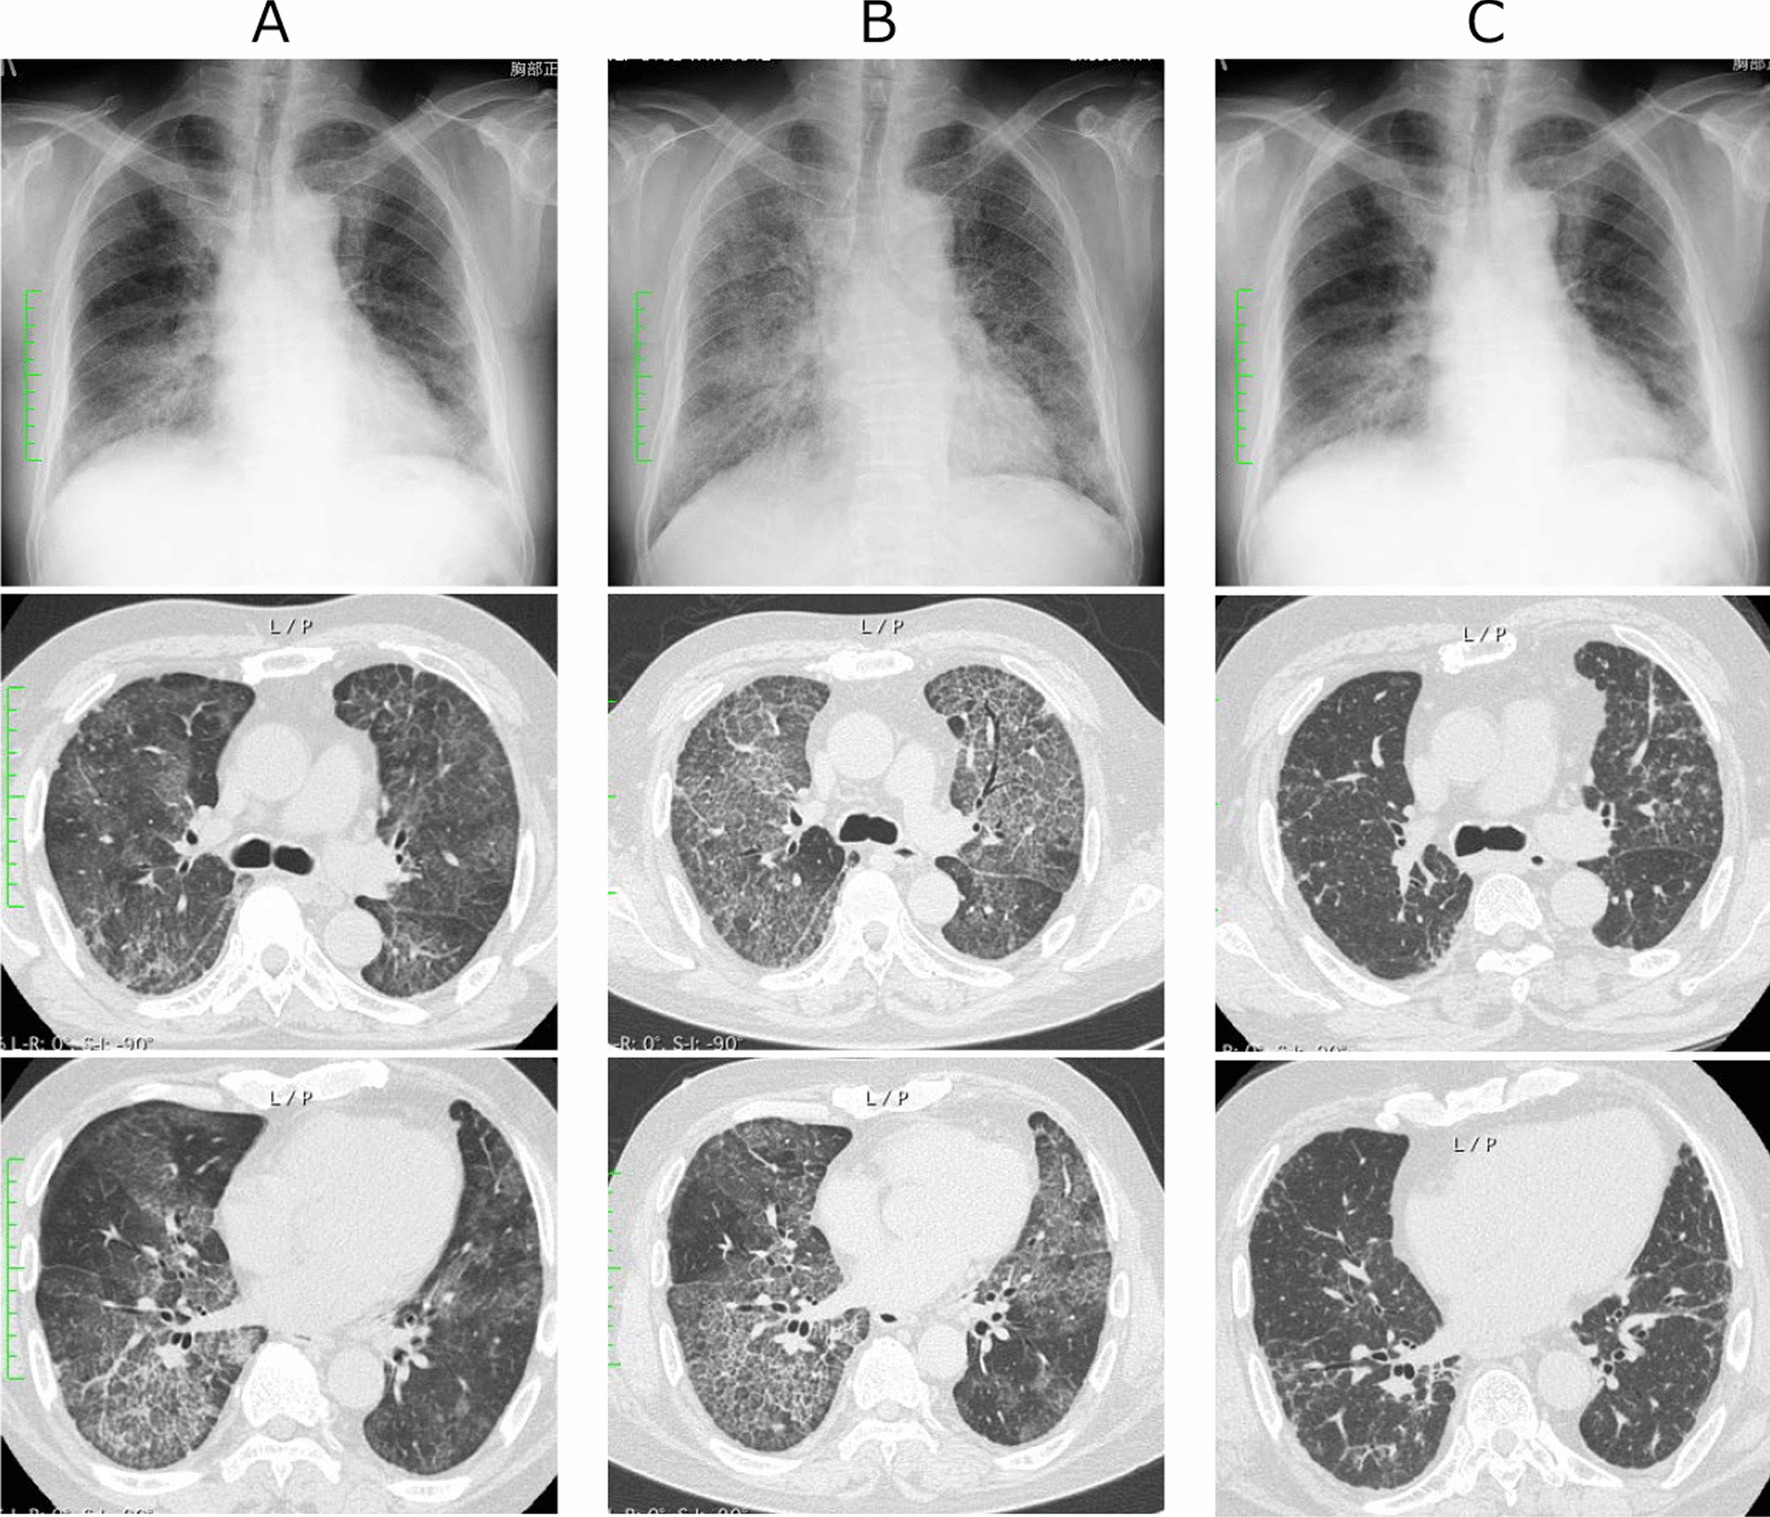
Chest radiography and computed tomography findings. First visit to the previous hospital, 2 months before referral to our hospital (A). At the time of referral to our hospital, the reticular shadows were enlarged on chest radiography and the crazy paving appearance was enlarged and well-defined on chest computed tomography (B). One year later, the chest imaging findings have improved following the reduction and discontinuation of steroids and immunosuppressive agent and repeated total lung lavage under general anesthesia (C)
